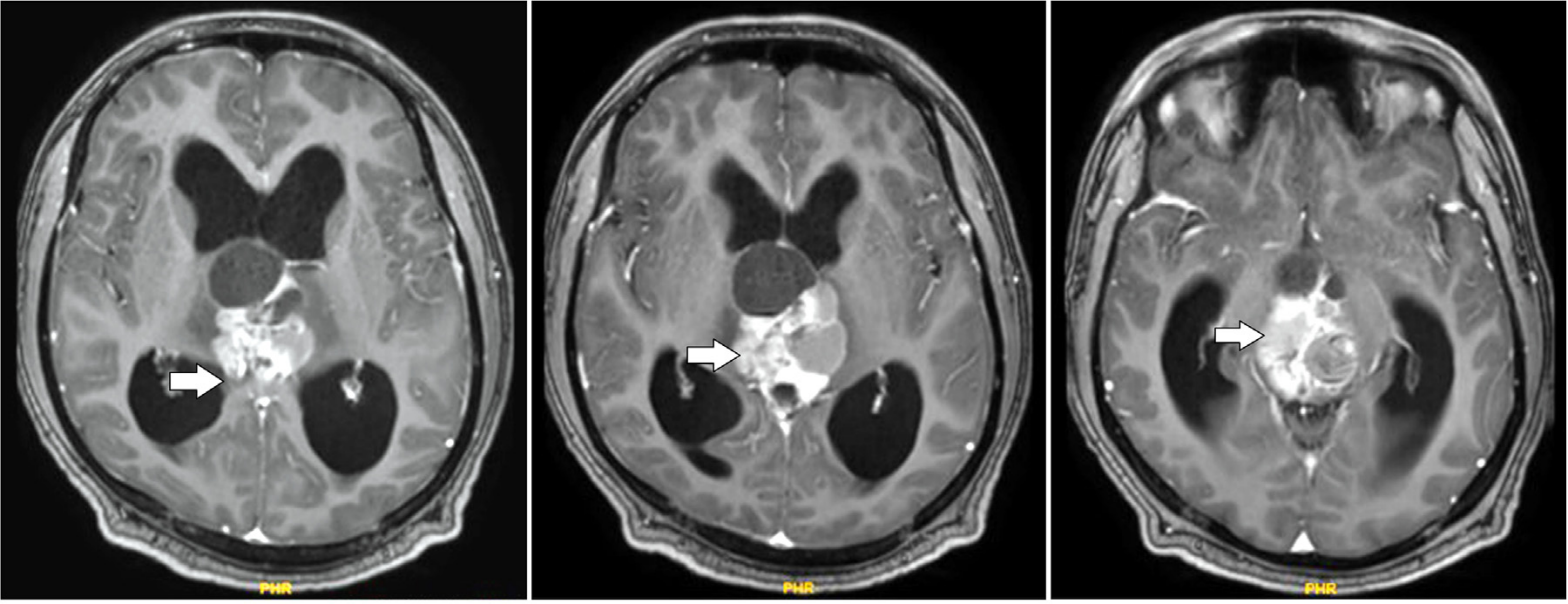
Preoperative T1 contrast MRI axial images showing the tumor in the pineal region.
